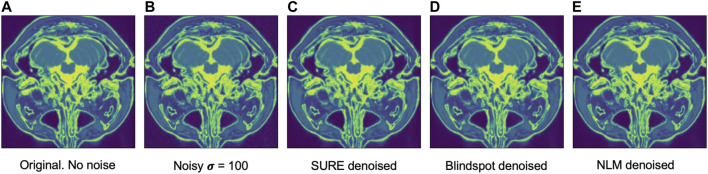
Example of denoised brain MRI for σ=100. The example image is the middle slice from one of the subjects. In this case, this is the PSNR for every method for this particular subject. (A) Original image, no noise added—(B) Noisy image—(C) SURE PSNR = 38.130—(D) Blindspot PSNR = 39.072—(E) NLM PSNR = 37.108.
